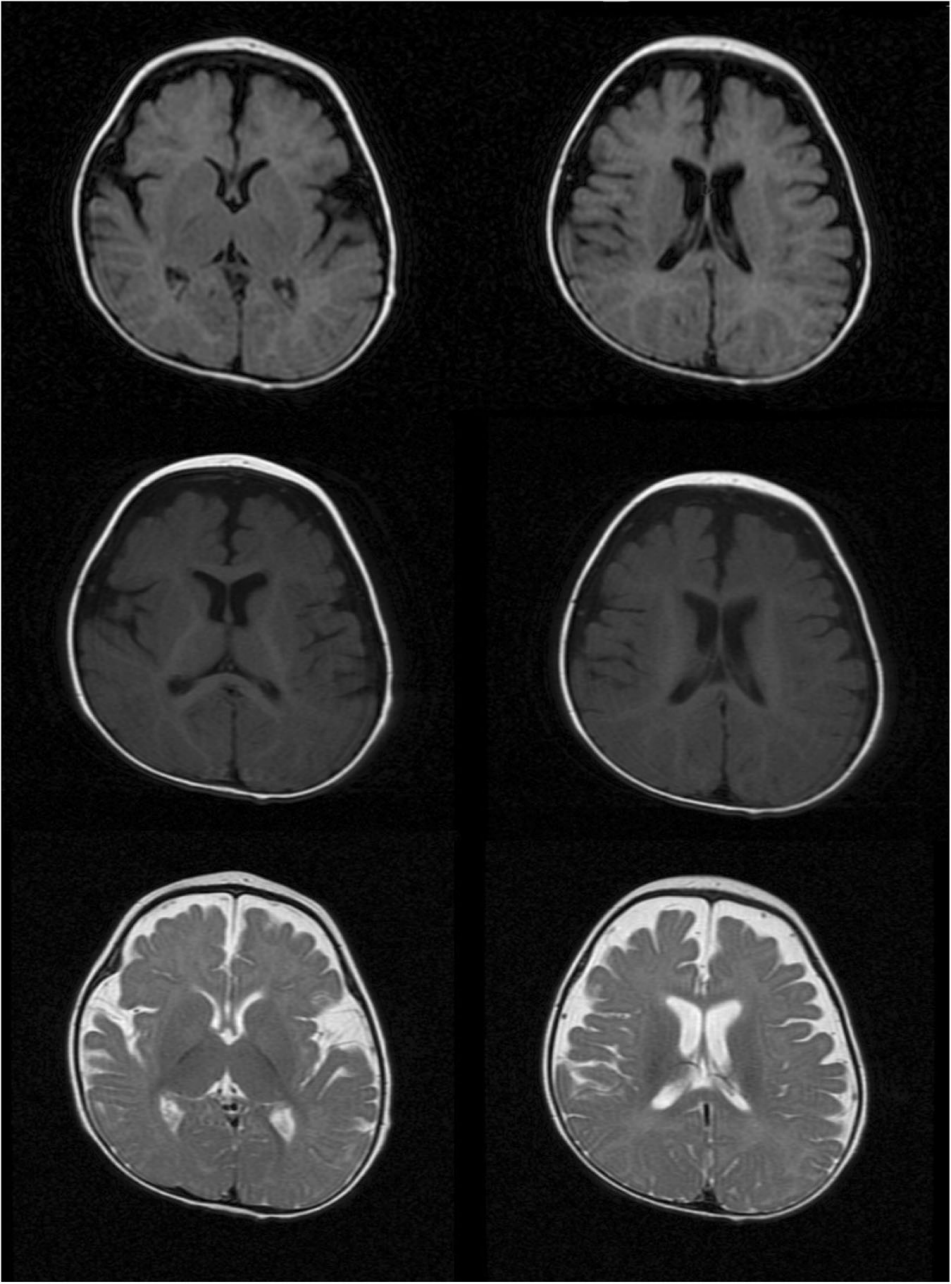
Brain MRI of the proband. Magnetic resonance imaging suggests mild cerebral atrophy (conducted at 6 months of age).
